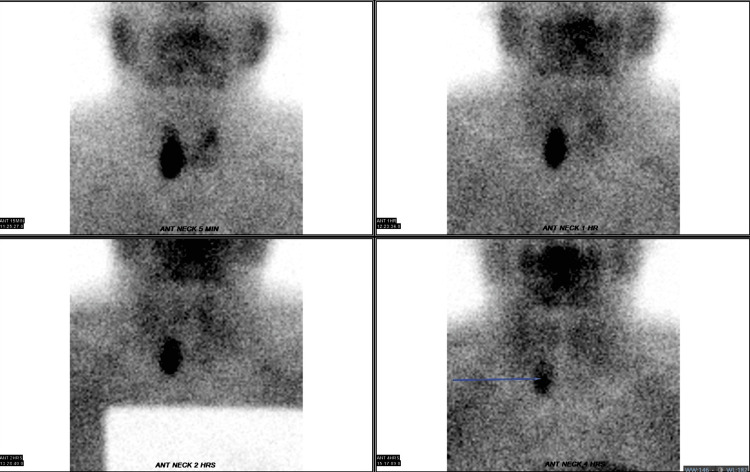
Parathyroid scintigraphy showing a lesion suggestive of functioning parathyroid adenoma at the inferior pole of the right thyroid lobe (blue arrow).
